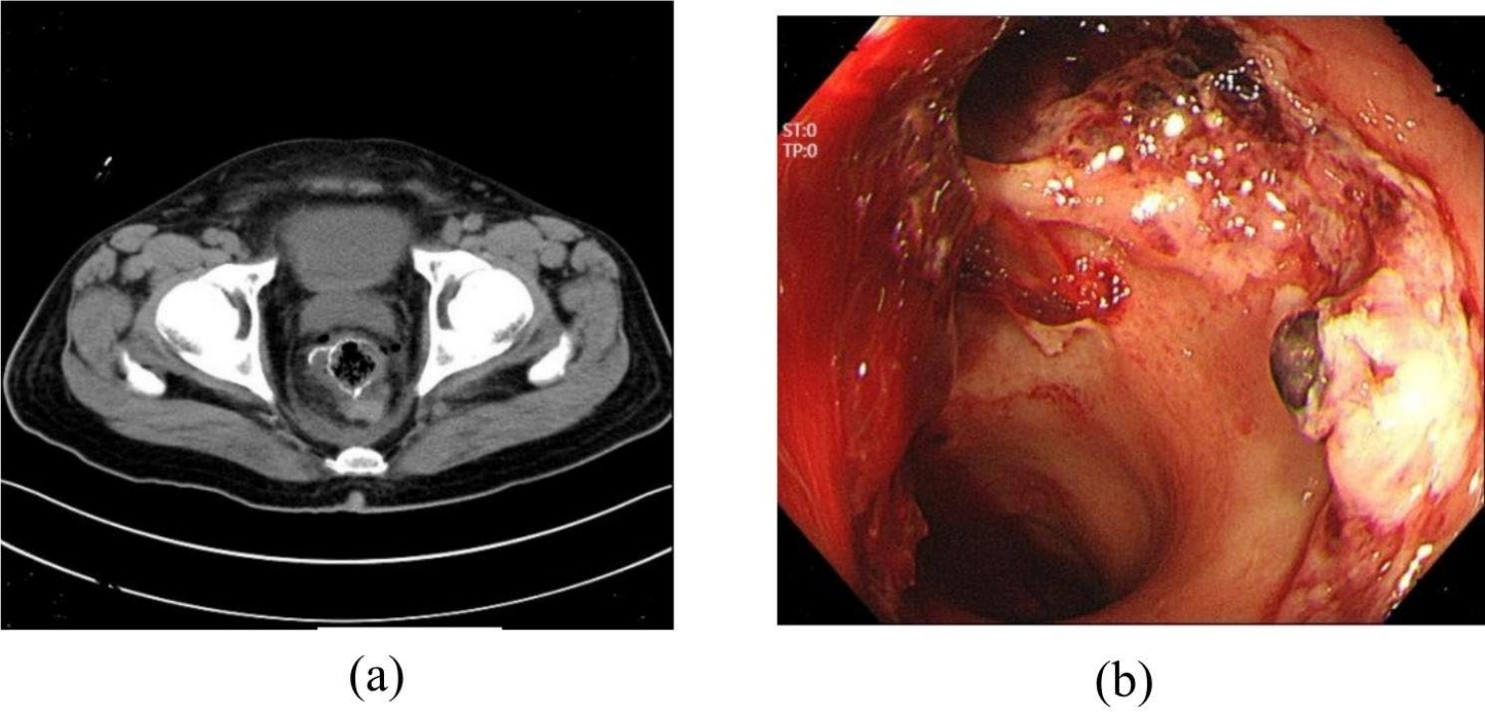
Image of AL. (a) Computed tomography scan of the pelvis: air bubbles and fluid around the rectal anastomosis; (b) Colonoscopy: rectal anastomotic defect
